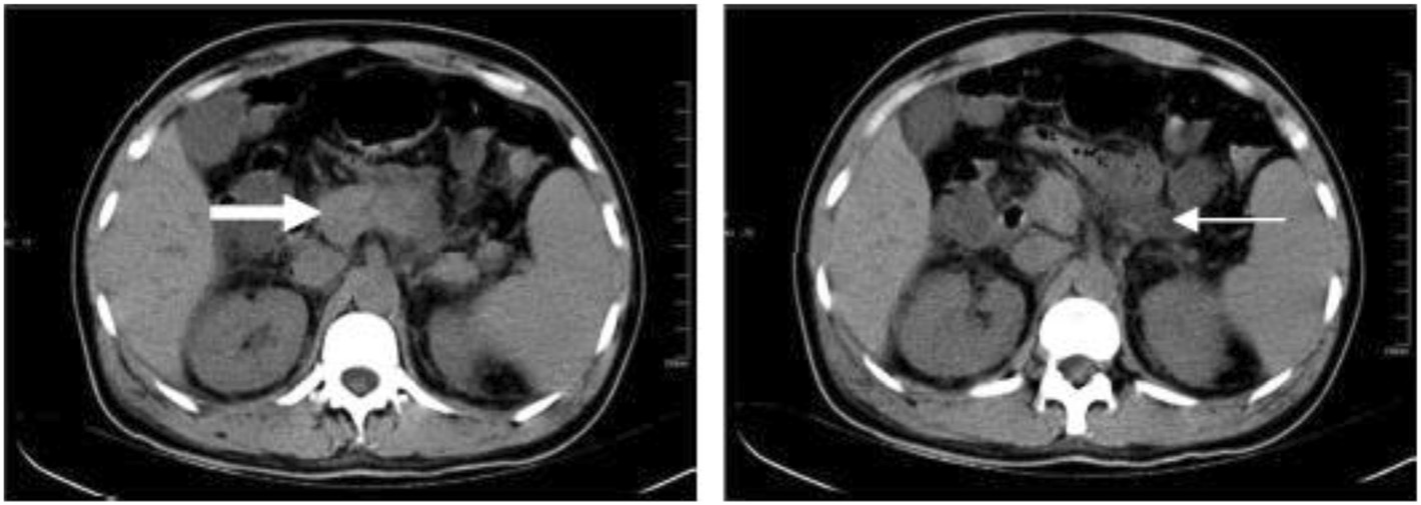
Abdominal CT images of a 41 year-old man with HFRS complicated with SAP (case 1). Edema of the pancreas (thick white arrow) and peripancreatic fluid collection (thin white arrow) are visible.
